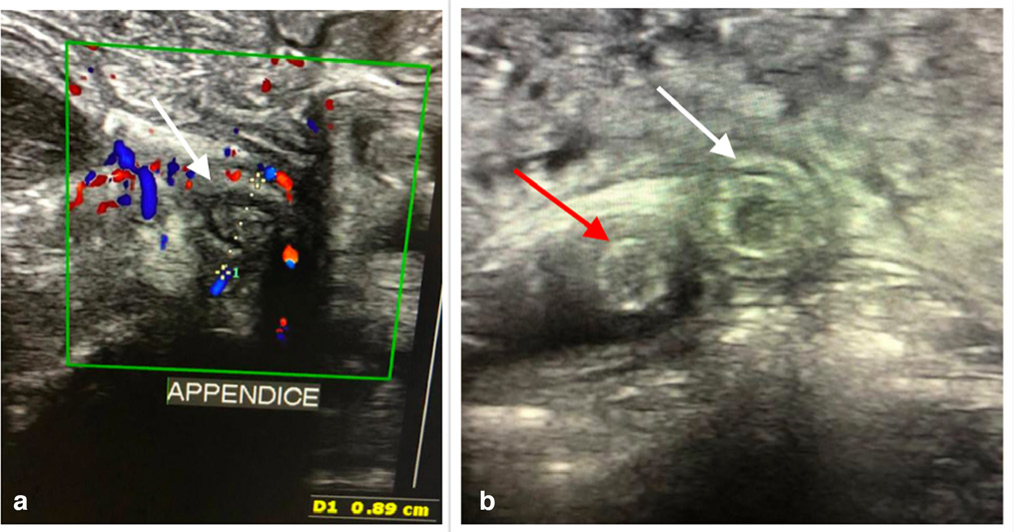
Axial view of the right iliac fossa with the high-frequency probe (A)appendix increased in diameter measuring 8.9mm (white arrow) with a dedifferentiated wall and hyper vascularized on color Doppler (B) double target-sign corresponding to the appendix (white arrow) and the Ascaris in contact (red arrow).
